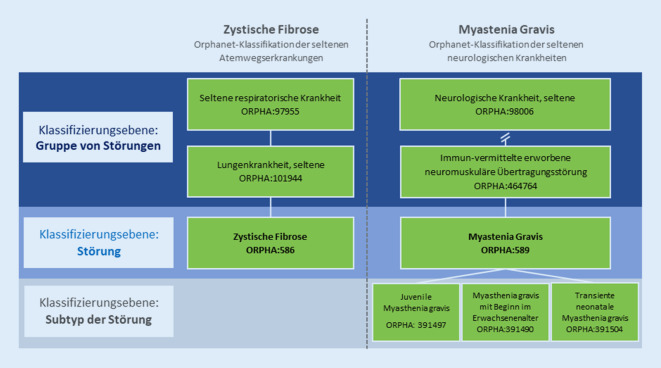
Die Einträge der Orphanet-Datenbank sind mit weiteren Terminologien und Kodiersystemen gemappt, so dass eine Interoperabilität zwischen verschiedenen Datensystemen ermöglicht wird. Das Mapping umfasst systematische Zuordnungen u. a. zu OMIM^1^, ICD-10-WHO^2^, UMLS^3^, SNOMED CT^4^, aber auch Thesauri, wie z. B. MeSH^5^ und MedDRA^6^. Alle Dateien der Orphanet-Terminologie inklusive Verweisdaten sind öffentlich und zum Download frei verfügbar auf der Online-Plattform Orphadata.org [5]. Die aktuell verfügbare Datei (ORPHA NOMENCLATURE PACK, Juli 2021) enthält 6165 aktive ORPHAcodes, die eine Kodierung von SE auf der von Orphanet definierten Ebene der Störung ermöglichen.
